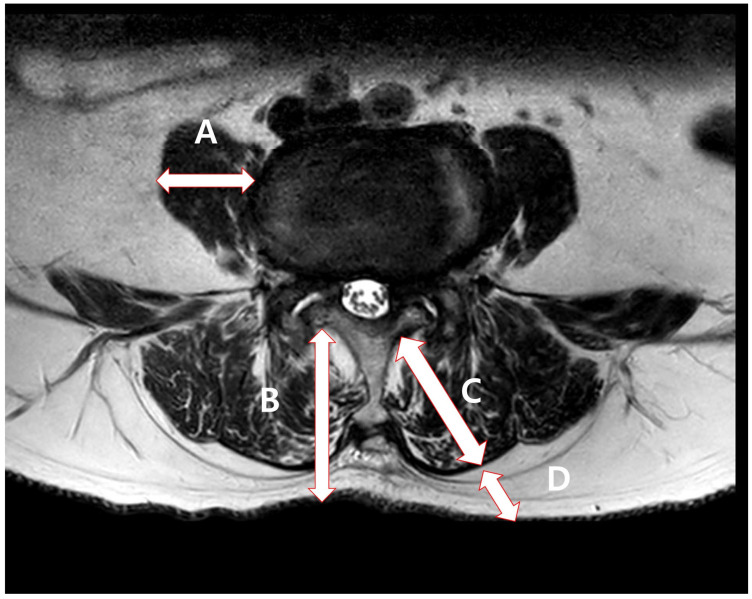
Measurement of soft tissue thickness. Soft tissue thickness was measured using preoperative MRI axial cut at the surgical level. A: Psoas muscle thickness, B: interlaminar thickness, C: Dorsal extensor muscle thickness, D: subcutaneous fat thickness.
